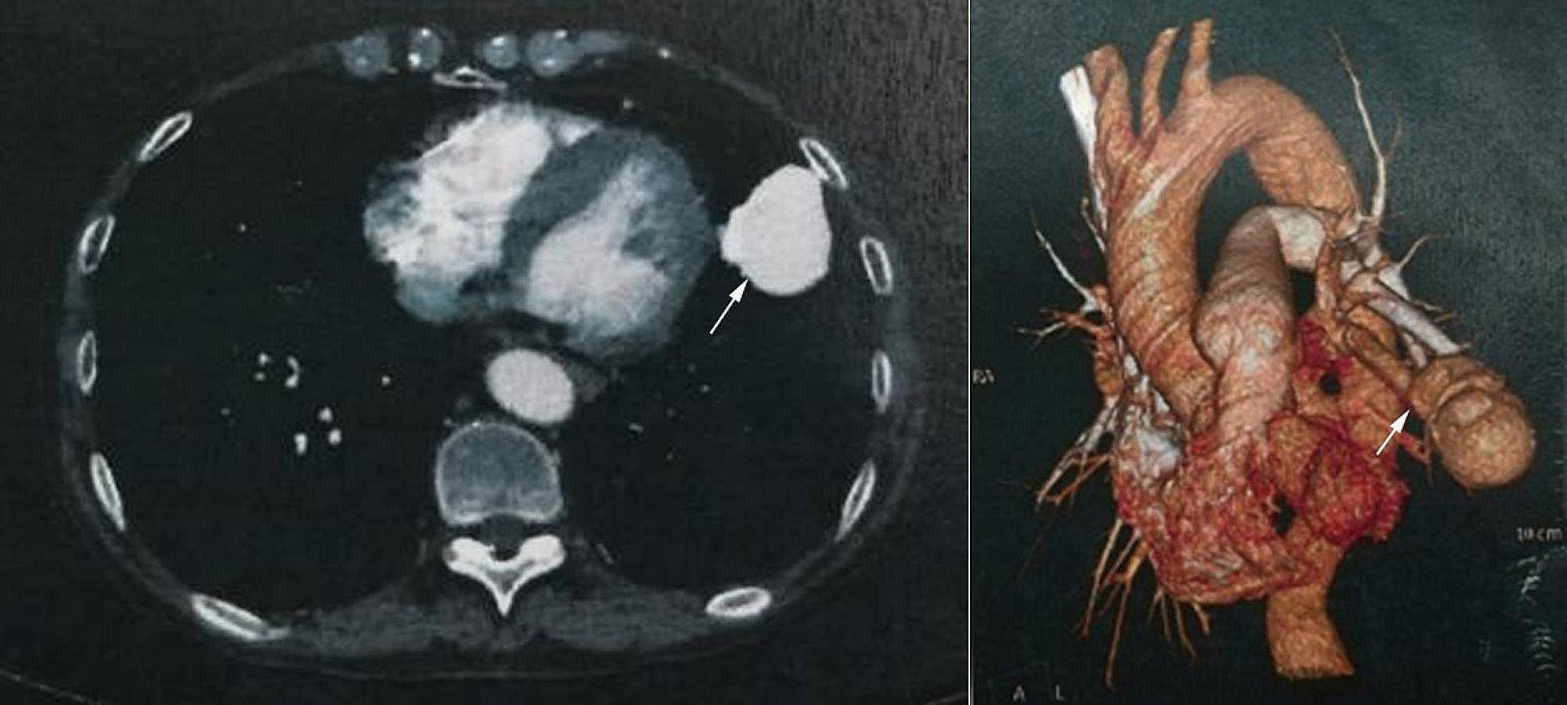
Pulmonary artery CTA showing abnormal density shadow with size about 4.7 cm×2.4 cm in the parahilar region of the left upper lobe tongue segment, considering pulmonary arteriovenous fistula
